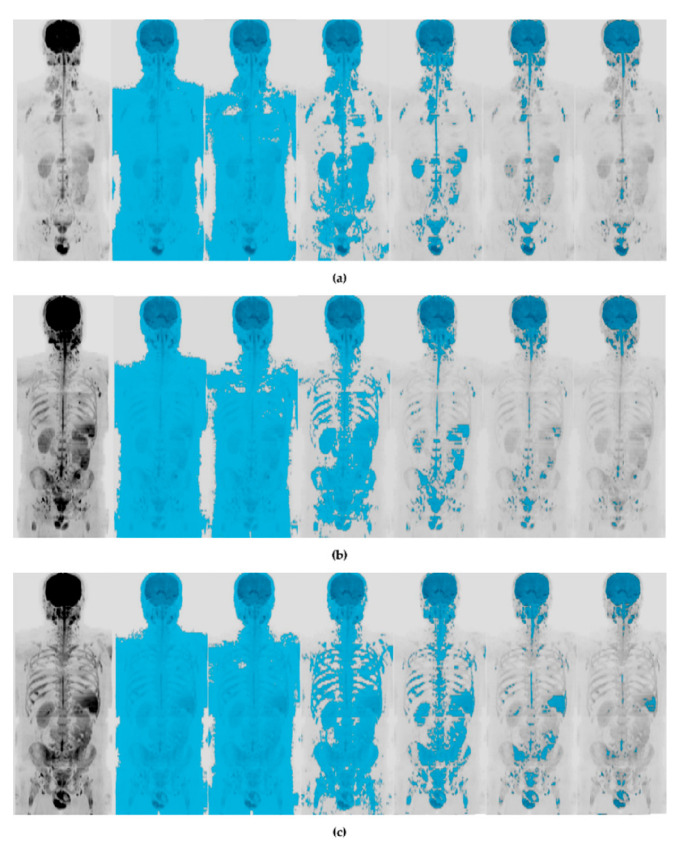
(a) Maximum intensity projection (MIP) of WB-DWI images at b800 at baseline (T0) and its relative masks at 5%, 10%, 20%, 40%, 60%, and 80%. (b) Maximum intensity projection (MIP) of whole-body DWI (WB-DWI) images at b800 at interim (T1) and its relative masks at 5%, 10%, 20%, 40%, 60%, and 80%. (c) Maximum intensity projection (MIP) of WB-DWI images at b800 at the EOT (T2) and its relative masks at 5%, 10%, 20%, 40%, 60%, and 80%. Images are from a patient showing complete metabolic response (according to Lugano evaluation) both at T1 and T2.
